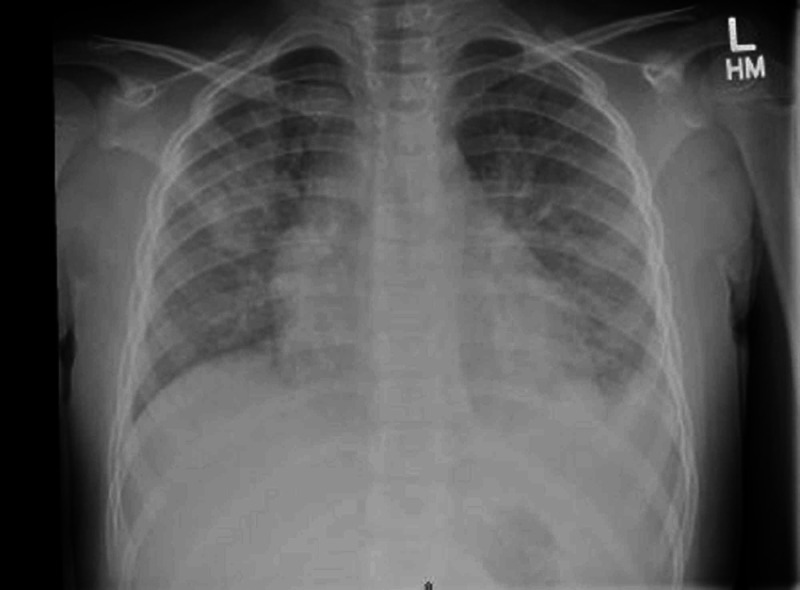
Chest x-ray on admission, showing a bilateral diffuse airspace opacities in the absence of multifocal pneumonia, pulmonary edema or acute respiratory distress syndrome
